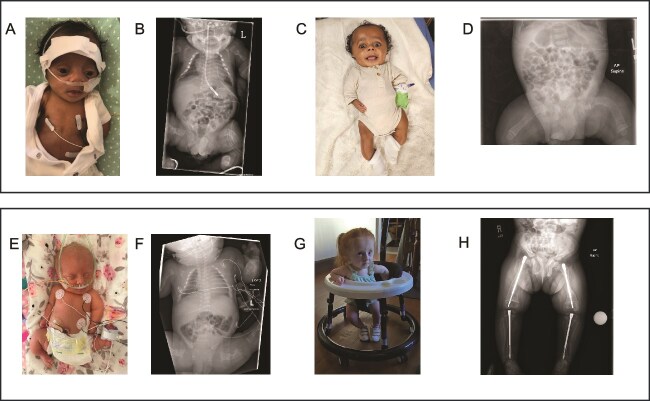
Clinical and radiographic progression of 2 infants with variants previously associated with lethal OI. (A) Patient 15 at 1 mo of age; clinical photograph, infant on nasal cannula. (B) Radiograph of patient 15 at 1 wk of age demonstrating demineralized bones, chest wall deformity, multiple healing rib fractures, broad capacious canals of the upper and lower extremities with thin crumpled cortices, multiple healing fractures and bowing deformities. (C) Patient 15 at 8 mo of age; clinical photograph during bisphosphonate infusion, infant awake, alert, spontaneously moving upper and lower extremities. Note limb bowing and blue sclera that are indicative of severe OI presentation. (D) Radiograph of patient 15 at 7 mo of age demonstrating persistent but improved demineralization, bilateral femoral bowing, thickening of cortices, pamidronate lines in the metaphyses. (E) Patient 2 at 1 d old, clinical photograph of infant on nasal CPAP, with feeding support via NG tube and umbilical venous catheter. Bowing and deformity in the lower extremities. (F) Radiograph of patient 2 at day of life 0 demonstrating umbilical venous catheter, thin gracile ribs, crumpled extremity bones with capacious canals, thin cortices and multiple healing fractures. (G) Patient 2 at 4 yr old; clinical photograph demonstrating ability to bear weight on lower extremities. (H) Radiograph of the lower extremities of patient 2 at 3 yr old demonstrating bilateral femoral and tibial telescopic intramedullary rods placed during realignment and intramedullary rodding procedures. With the rods in place, patient 2 is progressing in a stander and bearing weight through the bilateral lower extremities.
